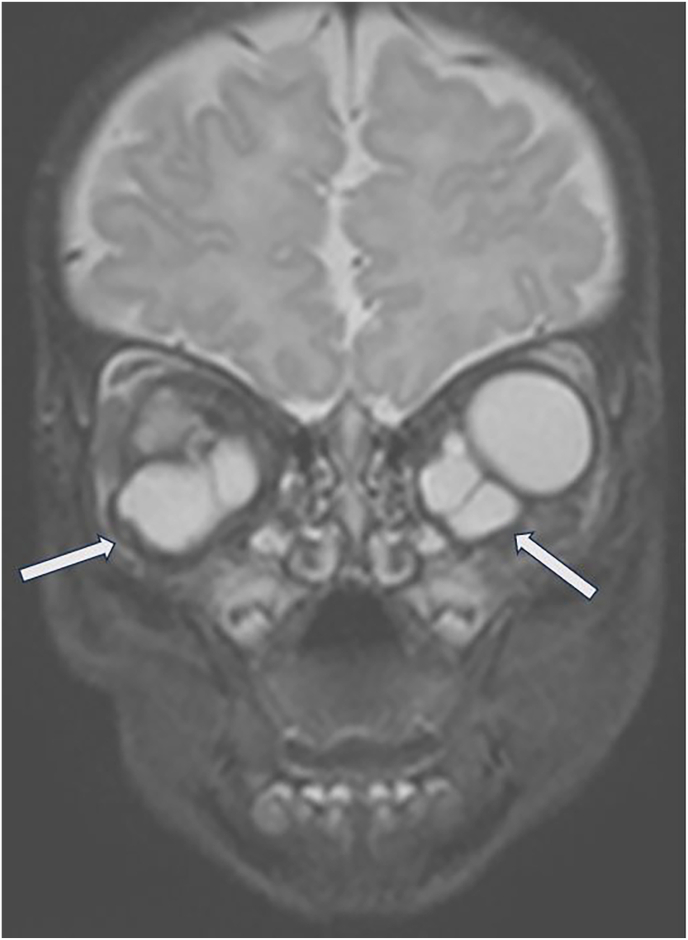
Coronal T2 weighted MR image of the orbits shows bilateral multiloculated retrobulbar cysts (white arrows).
